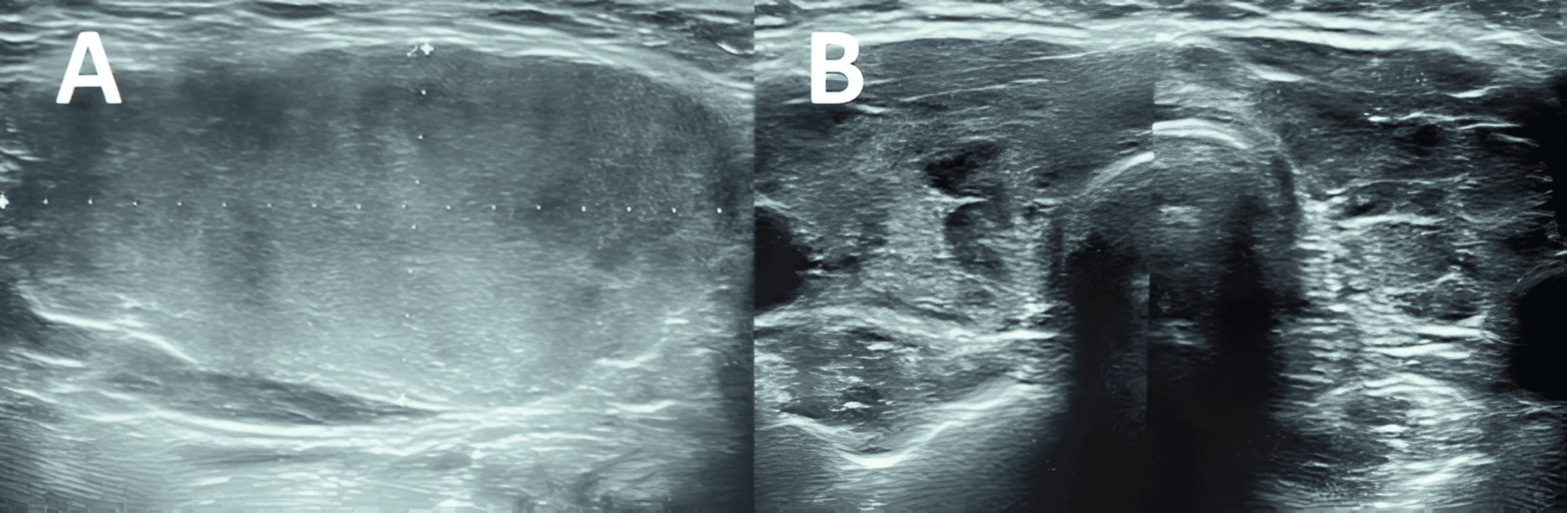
Ultrasound images showing a solid, non-homogeneous mass in the left submandibular gland, measuring 49.1 x 23 mm (A), and a goiter with diffuse hypoechoic areas without a clearly defined nodular image (B)
